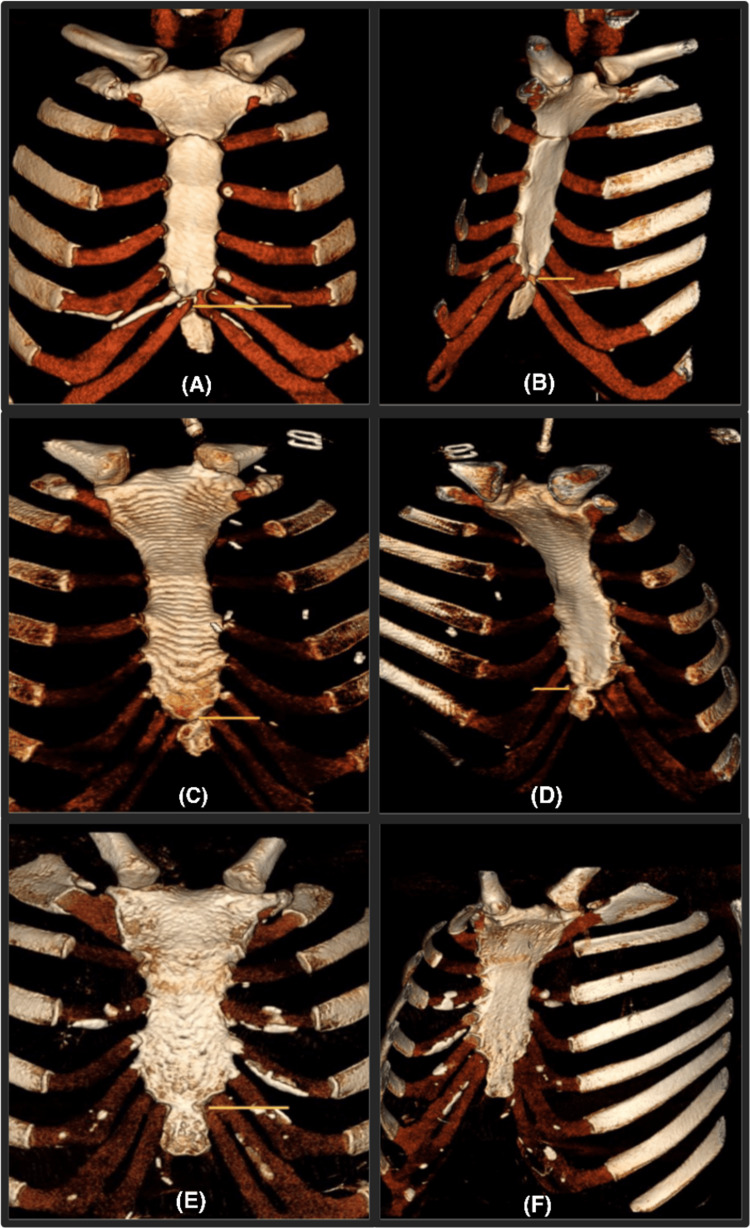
CT-3D volume rendered (VRT) image showing grades of xiphisternal joint ossification. (A) Unfused xiphisternal joint (grade 1-unfused joint), (B) posterior oblique view showing nonfusion at the joint, (C) partially fused xiphisternal joint (grade 2-partial fusion), (D) partial fusion of the xiphisternal joint more evident posteriorly, (E) completely fused xiphisternal joint (grade 3-complete fusion), and (F) posterior oblique view showing complete fusion of the joint. VRT: volume rendering technique.
